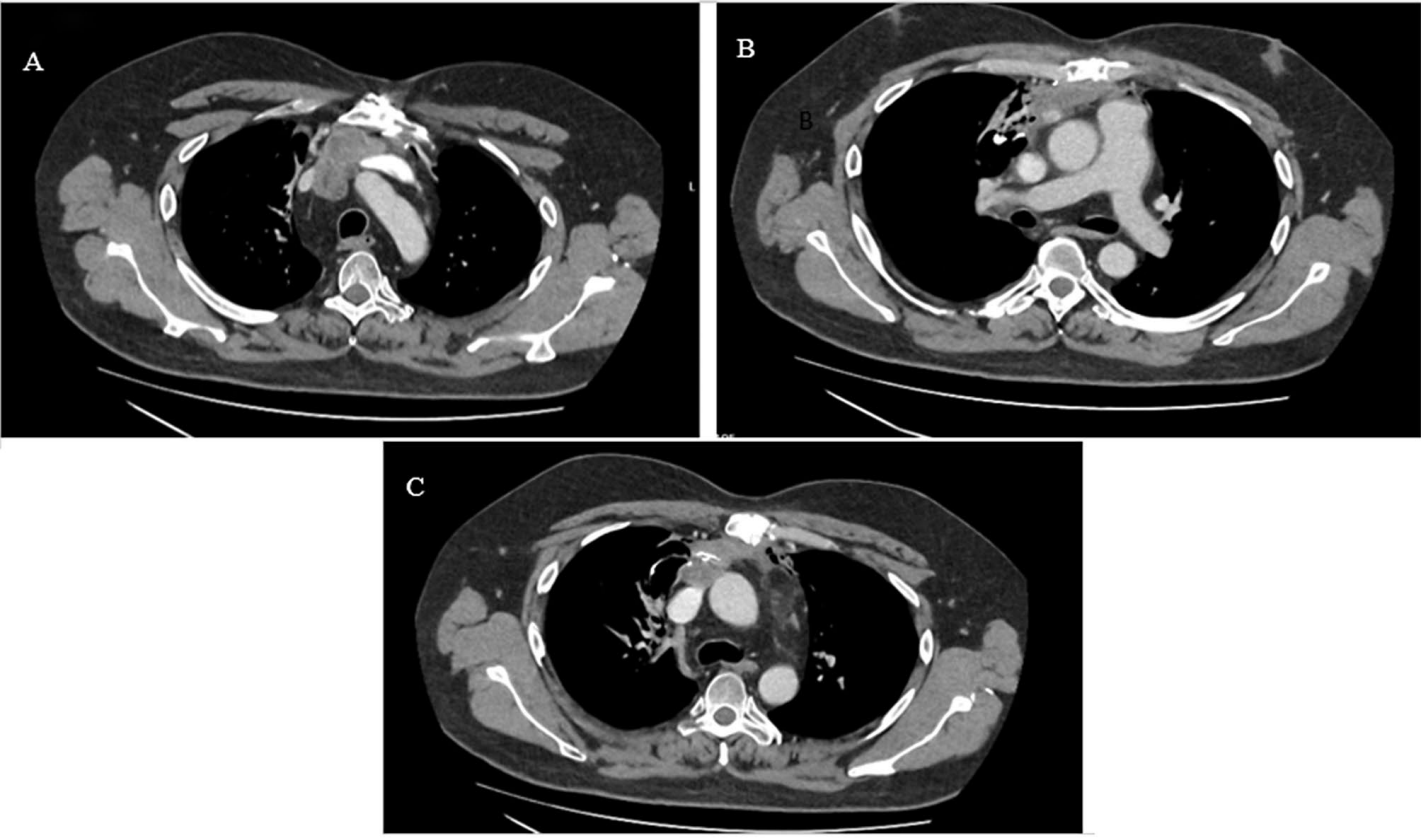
Contrast-enhanced CT axial section of anterior mediastinal mass post initial chemotherapy and 1st debulking operation (A). Mass at the level of the PA bifurcation and Right Ventricular outflow tract (RVOT) (B). Mass at the level of the carina (C)
